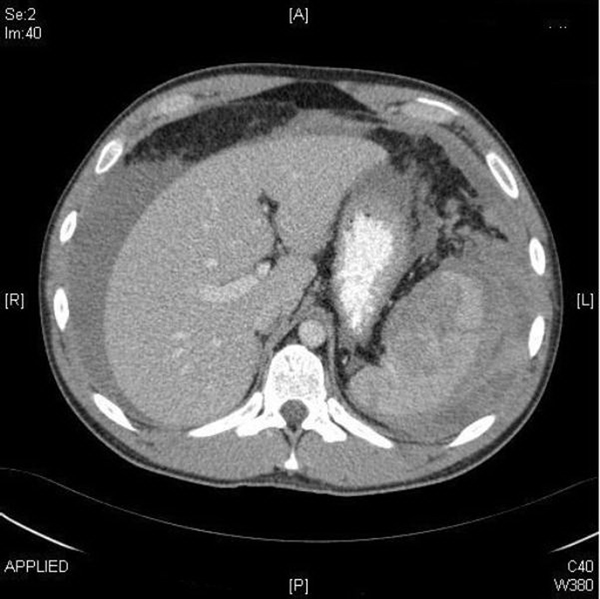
Transverse section on CT Abdomen showing free fluid (blood) and laceration of spleen.
